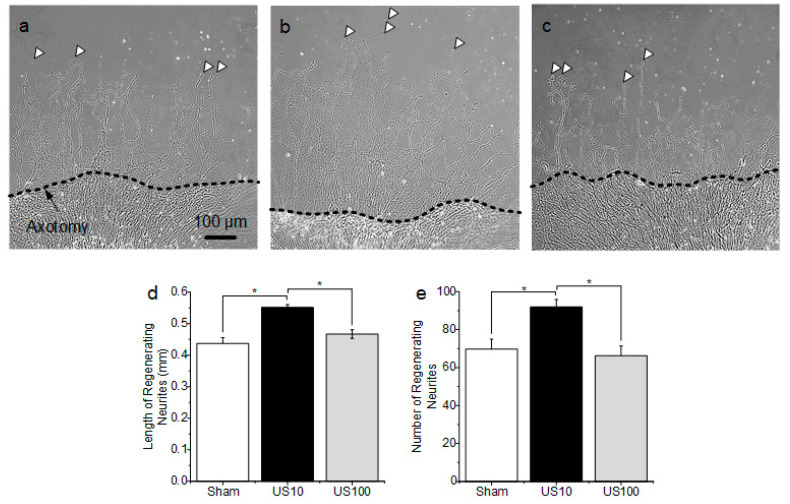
Evaluation of neurite regeneration of axotomized dorsal root ganglion (DRG) explants in response to ultrasound stimulation (US). (a) Representative images of regenerating neurites not treated with US (sham group). (b) Representative images of regenerating neurites treated with 10-Hz pulse-repetition frequency US (US10 group). (c) Representative images of regenerating neurites treated with 100-Hz pulse-repetition frequency US (US100 group). (d) Comparison of the lengths of regenerating neurites (*n* = 4). (e) Comparison of the numbers of regenerating neurites (*n* = 4). The data are expressed as the mean ± standard error of the mean (SEM); * *p* < 0.05.
